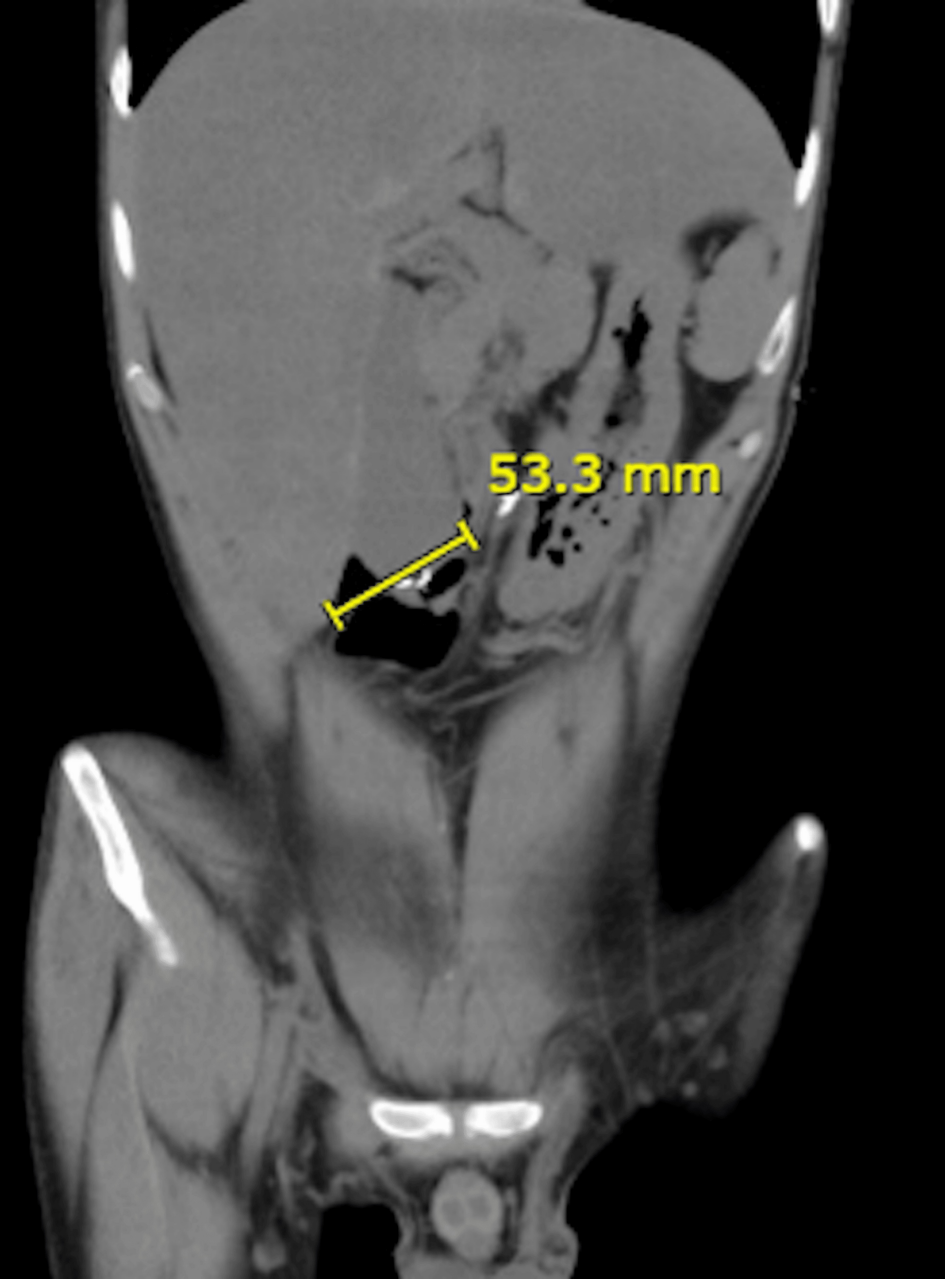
Abdominal CT scan of Case 1 This image shows a coronal view of the abdomen of a 27-year-old male patient with USBS following massive mesenteric ischemia and extensive intestinal resection. The scan reveals significant duodenal dilatation, with a diameter of up to 6 cm, and a complete absence of the small intestine, highlighting the limited absorptive surface necessitating surgical intervention. The yellow line measures the diameter of the significantly dilated duodenum. USBS: Ultra-short bowel syndrome
